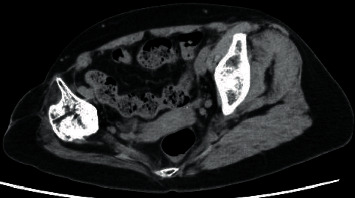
Computed tomography of the hip joint shows marked atrophy of the right gluteus medius muscle.
